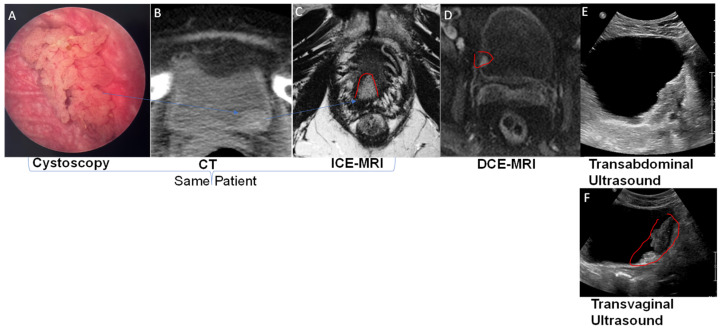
(A–C): A cystoscopically confirmed superficial tumor was imaged by CT and then by intravesical contrast-enhanced (ICE)-MRI after the instillation of the Gadolinium-based contrast agent GBCA and (Ferumoxytol) for positive and negative contrast, respectively, creating a clear demarcation of the bladder tumor (red circle) shape, size, and depth of penetration. (D–F): Dynamic contrast-enhanced magnetic resonance imaging (DCE-MRI) after intravenous injection of GBCA could image the NMIBC prior to TURBT, whereas the poor soft tissue resolution of CT is only good for estimating gross tumor volume, and the image resolution of transvaginal ultrasound (F) is superior to transabdominal ultrasound (E).
